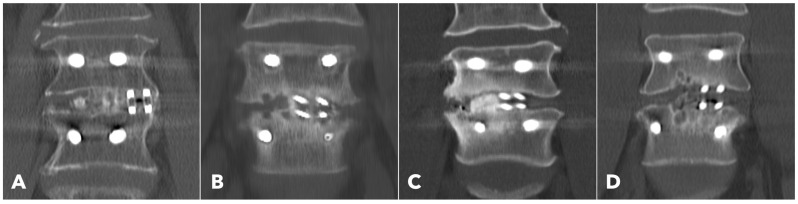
Postoperative 1-year CT reconstruction to evaluate the Bridwell fusion grade. (A) Grade I solid fusion with remodeling of the trabeculae. (B) Grade II fusion with bridging bone formation and no radiolucency between the cages and the endplates. (C) Grade III fusion with radiolucency between the bone graft and endplate. (D) Grade IV fusion with resorption of the bone graft.
